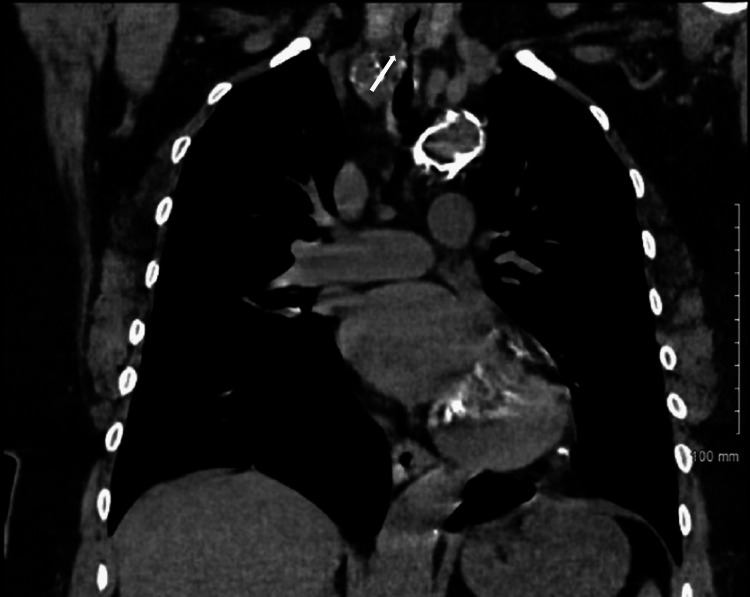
Coronal view of computed tomography scan of chest without contrast showing subglottic concentric tracheal stenosis measuring 2.6 cm in length
